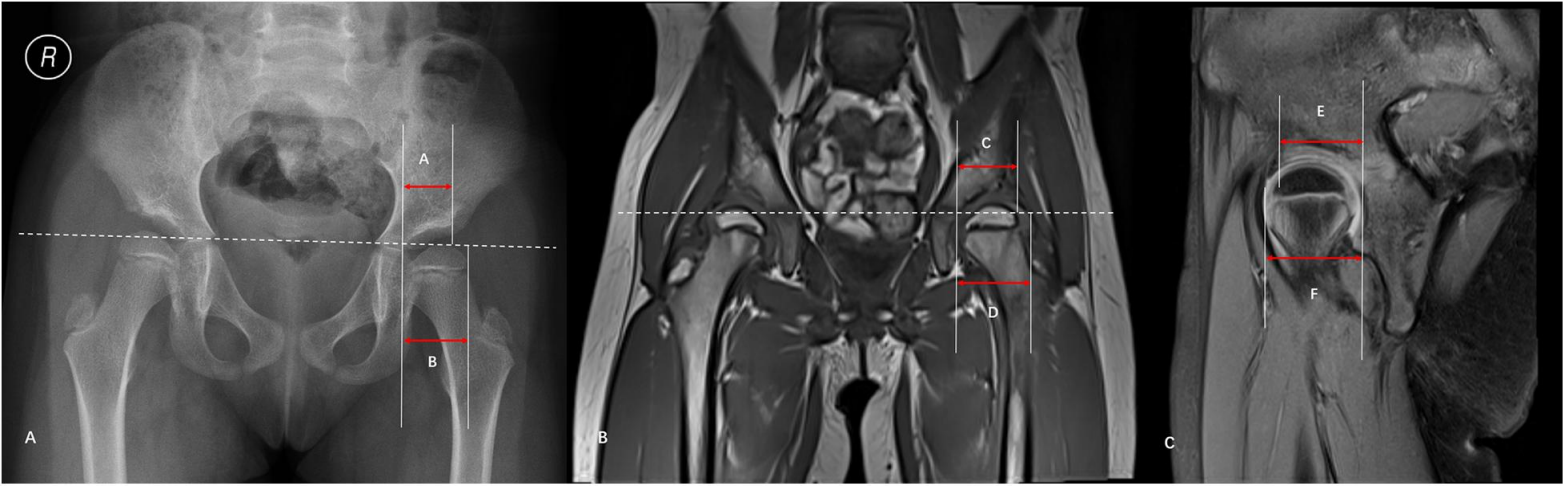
Measurement of the osseous and cartilaginous acetabular head indices in a 5-year-old girl with left developmental dysplasia of the hip (DDH) and grade II hip function at the time of imaging. The patient had undergone an open reduction of the left hip at 1.5 years of age. The images (A–C) demonstrate the measurement methods, yielding an osseous Acetabular Head Index (AHI) of 74.6%, a Lateral Cartilaginous AHI (L-CAHI) of 81.2%, and an Anterior Cartilaginous AHI (A-CAHI) of 87.1%. (A) Anteroposterior (AP) radiograph. A Is the distance from the medial edge of the femoral head to the lateral edge of the acetabular sourcil, and B is the maximum diameter of the femoral head. AHI = A/B × 100%. (B) Coronal T1-weighted image (T1WI). C Is the distance from the most medial edge of the femoral head cartilage to the most lateral edge of the acetabular cartilage, and D is the maximum diameter of the femoral head. L-CAHI = C/D × 100%. (C) Oblique sagittal proton density-weighted image with fat suppression. E is the distance from the most posterior edge of the femoral head cartilage to the most anterior edge of the acetabular cartilage, and F is the maximum anteroposterior diameter of the femoral head A-CAHI = E/F × 100%.
